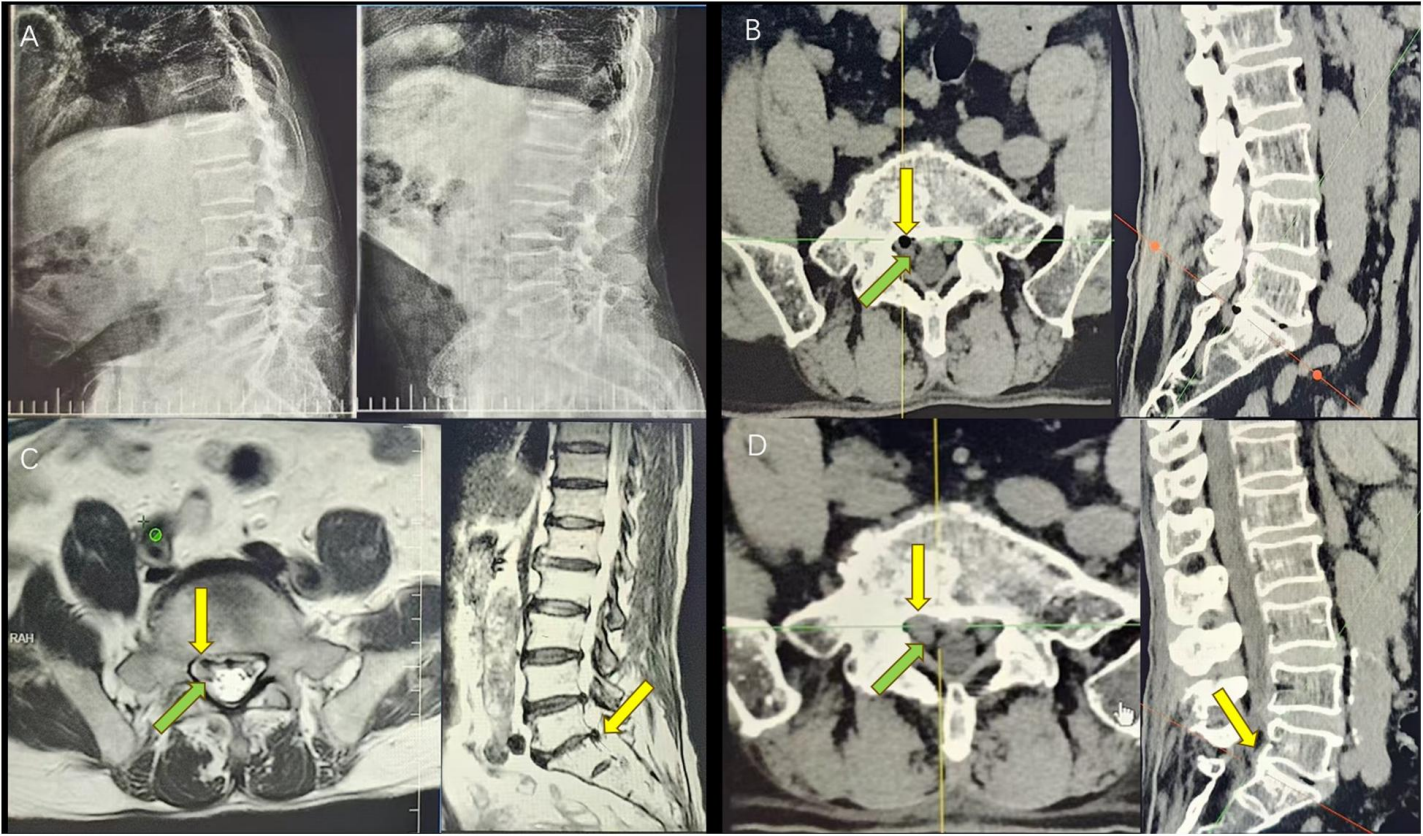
(A) Preoperative DR shows that the height of the L5/S1 intervertebral space is reduced and the vertebral body is still stable. (B) Preoperative CT shows an L5/S1 disc vacuum sign, right disc herniation with vacuoles (the yellow arrow indicates the location of the herniated disc), and a compression of the corresponding nerve root (the green arrow indicates the location of the compressed nerve root emanation). (C) Preoperative MRI shows a T1/T2 low hyperdensity shadow (the yellow arrow indicates the location of the herniated disc) and the corresponding compressed nerve root (the green arrow indicates the location of the compressed nerve root emanation). (D) Postoperative CT suggesting disappearance of the cyst (the yellow arrow suggests the location of the original cyst) and relaxation of the nerve root (the green arrow shows nerve root relaxation).
